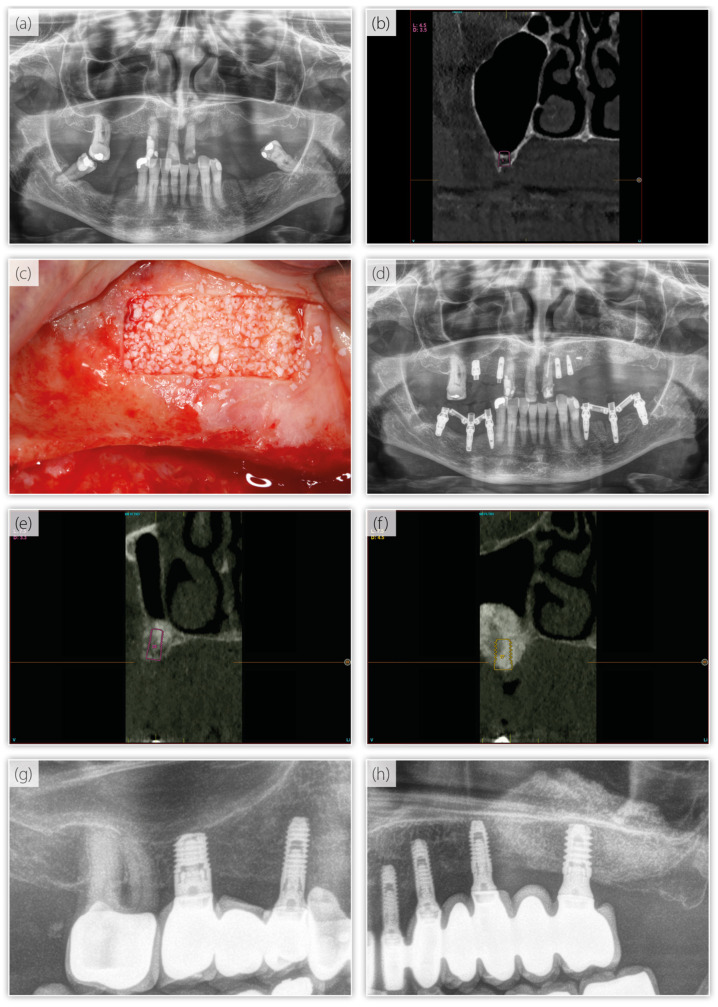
(a) Panoramic radiograph at the baseline. (b) Implant planning for the first quadrant, featuring a short implant to be placed directly. (c) Image of the lateral maxillary sinus elevation in the second quadrant. (d) Radiograph after the placement of the dental implants. (e,f) Implant planning in the sinus elevation area following graft integration. (g,h) Good clinical performance of all the implants.
